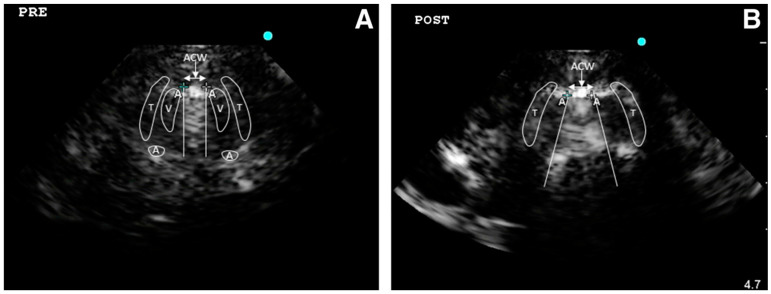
The imaging from laryngeal ultrasound demonstrates the air column width before (A) and after (B) endotracheal tube cuff deflation. After cuff deflation, the fan-shaped widening air column width obliterates surrounding structures such as vocal cords and arytenoid cartilage by acoustic shadow (T, thyroid cartilage; V, vocal cord; A, arytenoid cartilage). Reproduced with permission of Dr. Sutherasan from [16].
